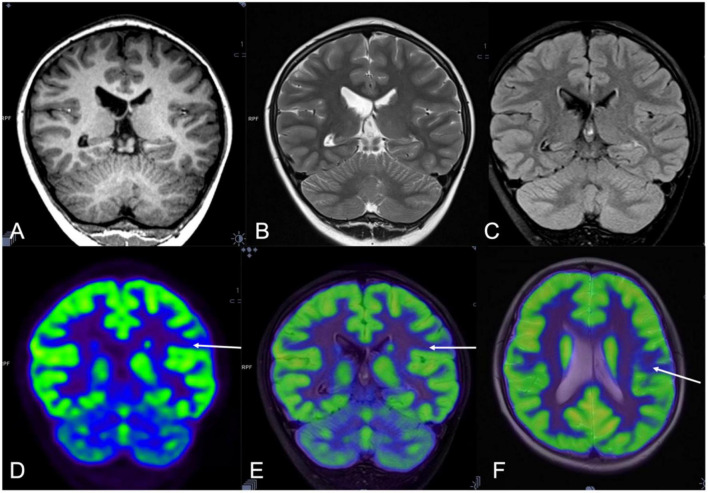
A 10-year-old man (patient #8) with refractory epilepsy for 3 years. Magnetic resonance (MR) imaging coronal T1 (A), T2 sequence (B), and Flair images (C) were negative. Positron emission tomography (PET) (D) and hybrid PET/MRI showed focal hypometabolic area in the left supramarginal gyrus (E,F). Combined with semiology and stereoelectroencephalography (SEEG) results, the left supramarginal gyrus was supposed as the seizure onset zone (SOZ) and stereoelectroencephalography-guided radiofrequency thermo coagulation (SEEG-guided RFTC) was performed. And the patient was seizure-free at the 47-month follow-up.
